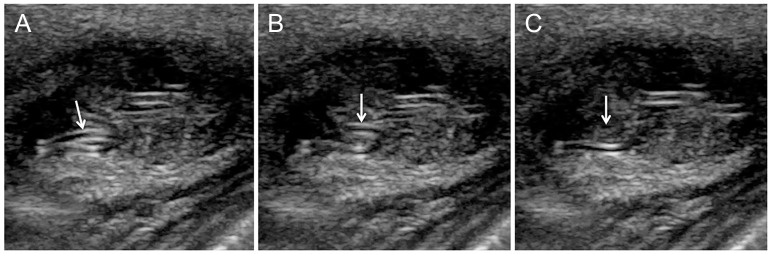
Images extracted from a short ultrasound video taken late with the motionless probe almost tangent to the skin plane, show that certain echogenic structures move and then disappear (arrowheads in A, B, and C), resulting from their spontaneous mobility.
